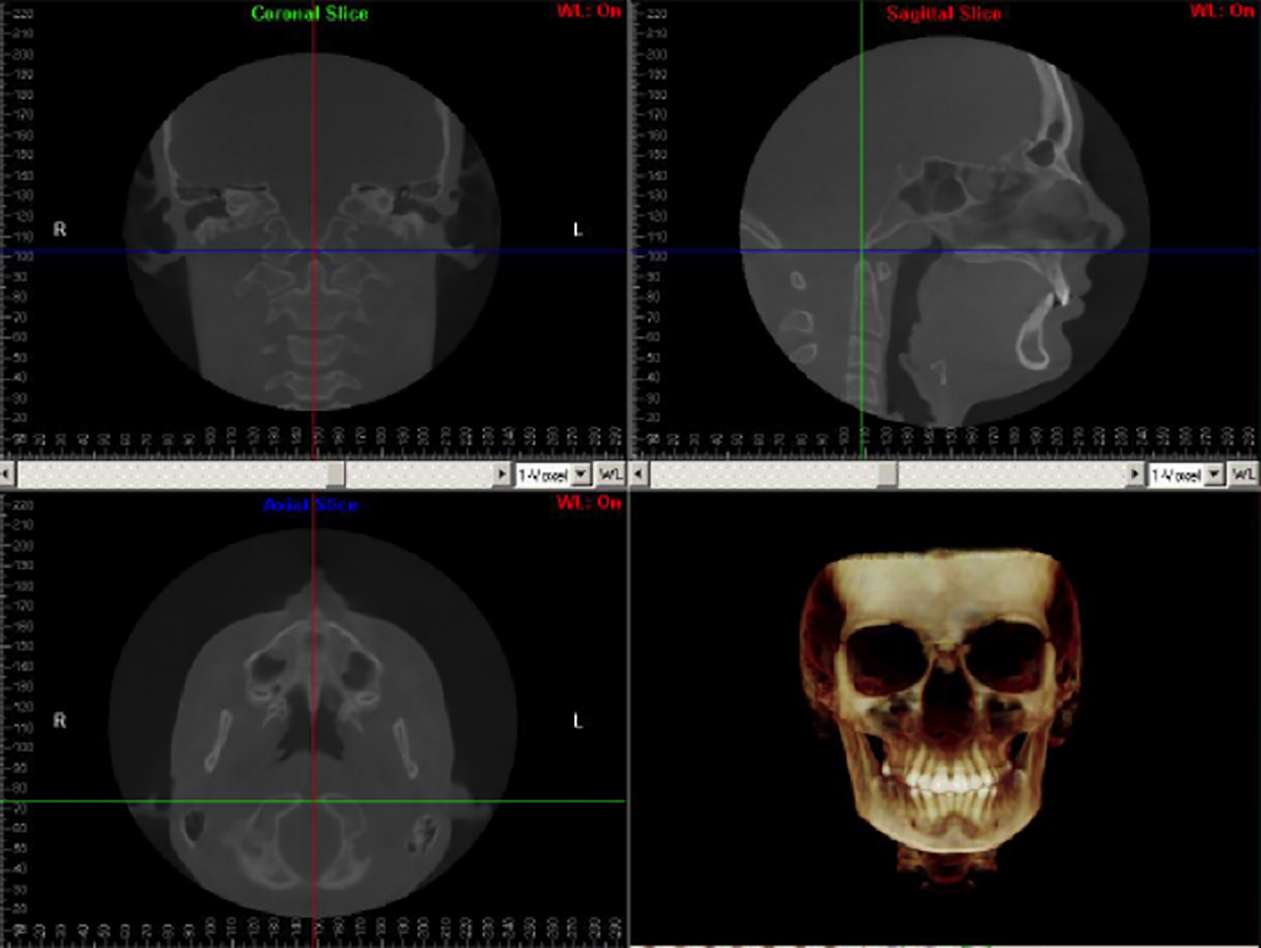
Mid-sagittal CBCT evaluation of spheno-occipital synchondrosis while the head in the default orientation.
